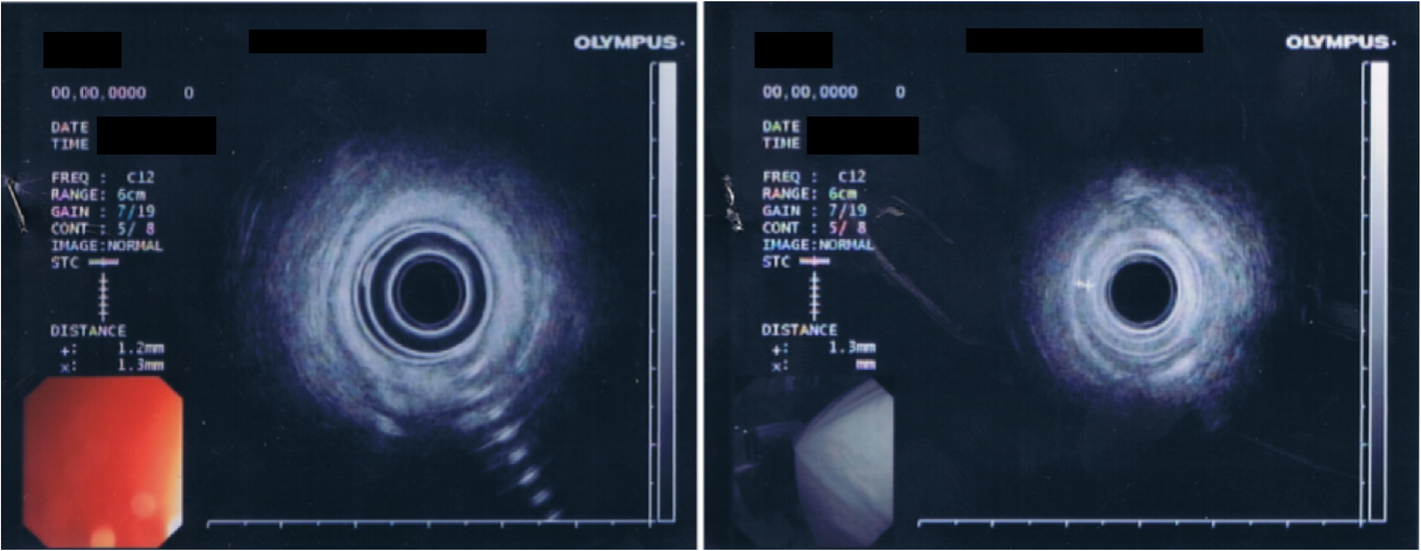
Transrectal endoscopic ultrasonography (TRUS). TRUS examination shows external anal sphincter echo structural normal. Internal anal sphincter without interruption of continuity, but of reduced thickness, about 1.3 mm measured at about 9 o’clock and 3 in correspondence of the middle part of the anal canal
